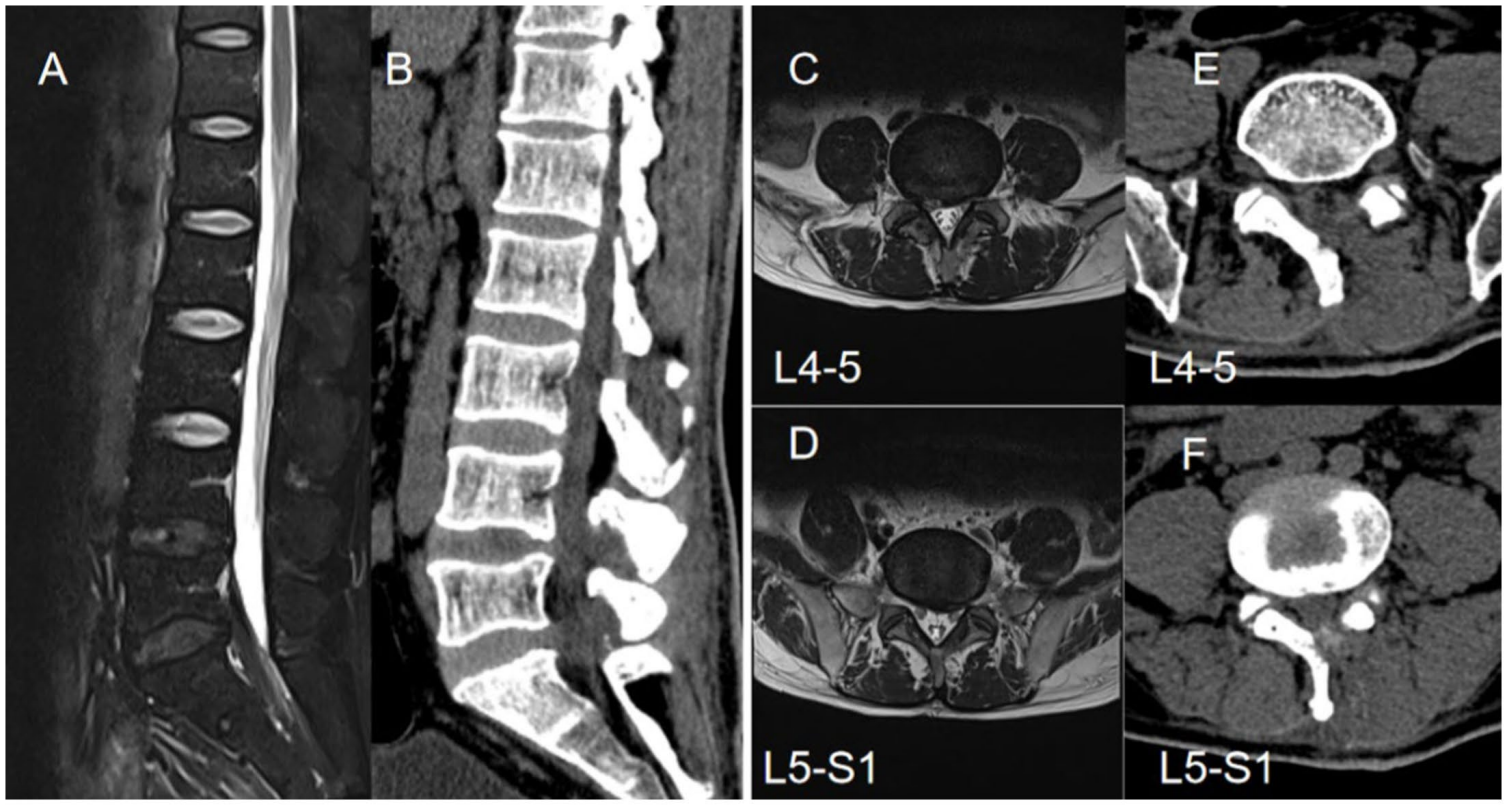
A 49-year-old male patient presented with L4-5 disc herniation and L5-S1 epidural lipomatosis, exhibiting clinical symptoms of pain and numbness in both lower limbs, with more severe manifestations on the right side (A,B). Preoperative axial and sagittal magnetic resonance imaging revealed L4-5 disc herniation and increased L5-S1 epidural fat, leading to spinal stenosis(C,D). Subsequent axial CT findings post-unilateral laminectomy demonstrated postoperative spinal canal widening on three-dimensional CT scan (E,F).
